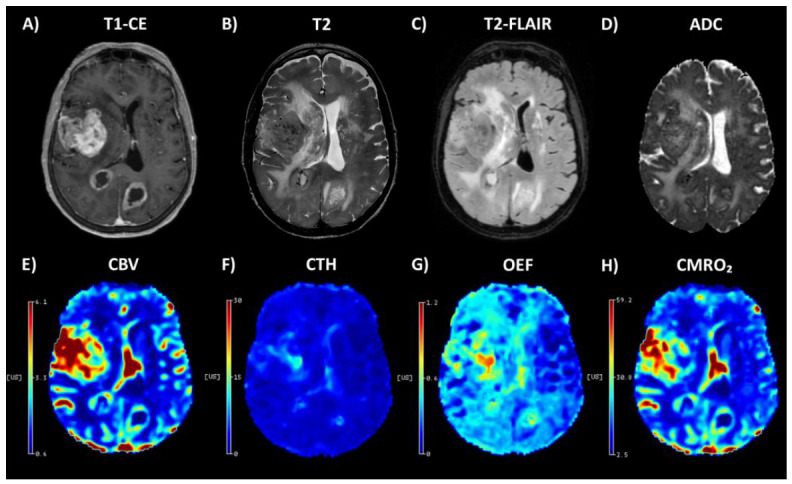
Representative preoperative MRI at 3 T with DSC-derived parametric maps in untreated *IDH*-wildtype glioblastoma (MGMT promoter unmethylated, TERT promoter wildtype; 3 T). (A) Post-contrast T1-weighted imaging (T1-CE), (B) T2-weighted imaging, (C) T2-FLAIR, (D) apparent diffusion coefficient (ADC) map, (E) cerebral blood volume (CBV) map, (F) capillary transit time heterogeneity (CTH) map, (G) oxygen extraction fraction (OEF) map, and (H) cerebral metabolic rate of oxygen (CMRO_2_) index map. Color bars indicate the relative scale of the map.
